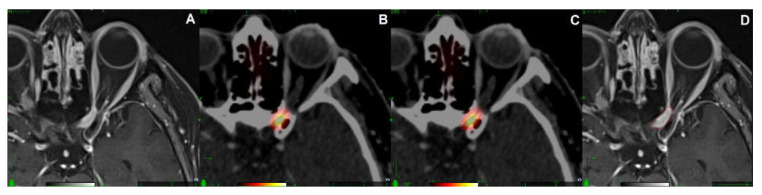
Radiosurgery contouring process: (A) Optic nerve sheath meningioma (ONSM) left eye difficult to see on MRI alone; (B) 68Ga-DOTATOC-PET/MRI co-registered with the planning CT demonstrates left canalicular location of ONSM; (C) initial tumor contouring based on the PET signal; (D) fine-tuning of the tumor volume using the MRI of the hybrid PET/MRI, no safety margin was added to generate the planning target volume (red line). MRI, magnetic resonance imaging, Ga68-DOTATOC, gallium-68-labeled (DOTA0-Phe1-Tyr3) octreotide; PET, positron emission tomography; CT, computer tomography.
